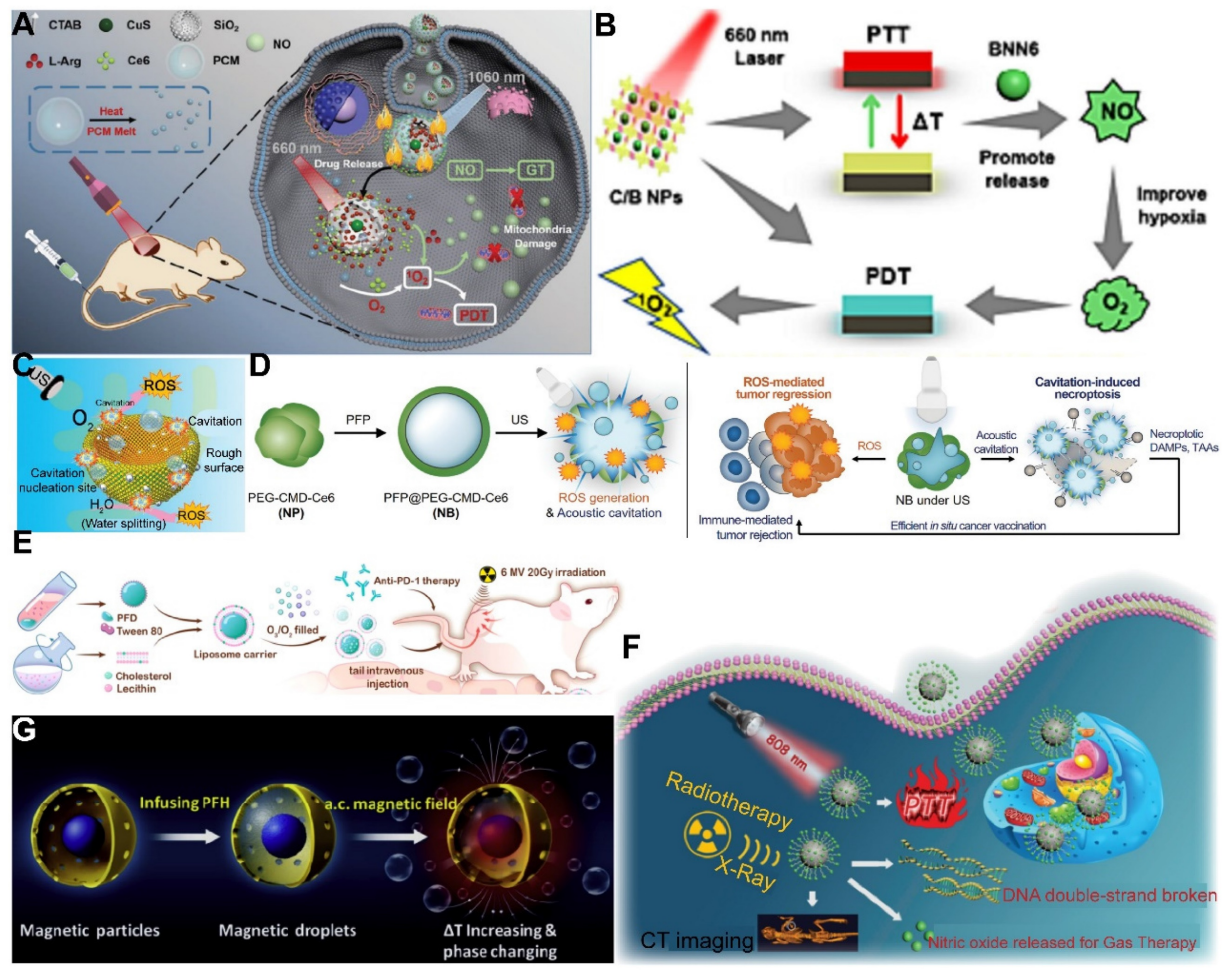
Exogenous stimuli-responsive gas delivery systems. (A) A NIR-responsive nanoplatform (CuS@SiO_2_-L-Arg@PCM-Ce6). Adapted with permission from 112, copyright 2021 Wiley-VCH. (B) A NIR-responsive nanoplatform (C/B@M NPs). Adapted with permission from 113, copyright 2024 Elsevier. (C) An US-responsive nanoplatform (APBN). Reproduced with permission from 115, copyright 2024 Xiahui Lin* et al.* (D) An US-responsive necroptosis-inducible NBs. Adapted with permission from 119, copyright 2020 Wiley-VCH. (E) An X-ray-responsive gas delivery system (O_3_/O_2__PFD@Liposome). Adapted with permission from 79, copyright 2022 Elsevier. (F) An X-ray-responsive gas delivery system (Bi-SNO NPs). Reproduced with permission from 124, copyright 2020 Royal Society of Chemistry. (G) A magnetic-responsive PFH-encapsulated MPs (MDs). Adapted with permission from 129, copyright 2017 Elsevier.
